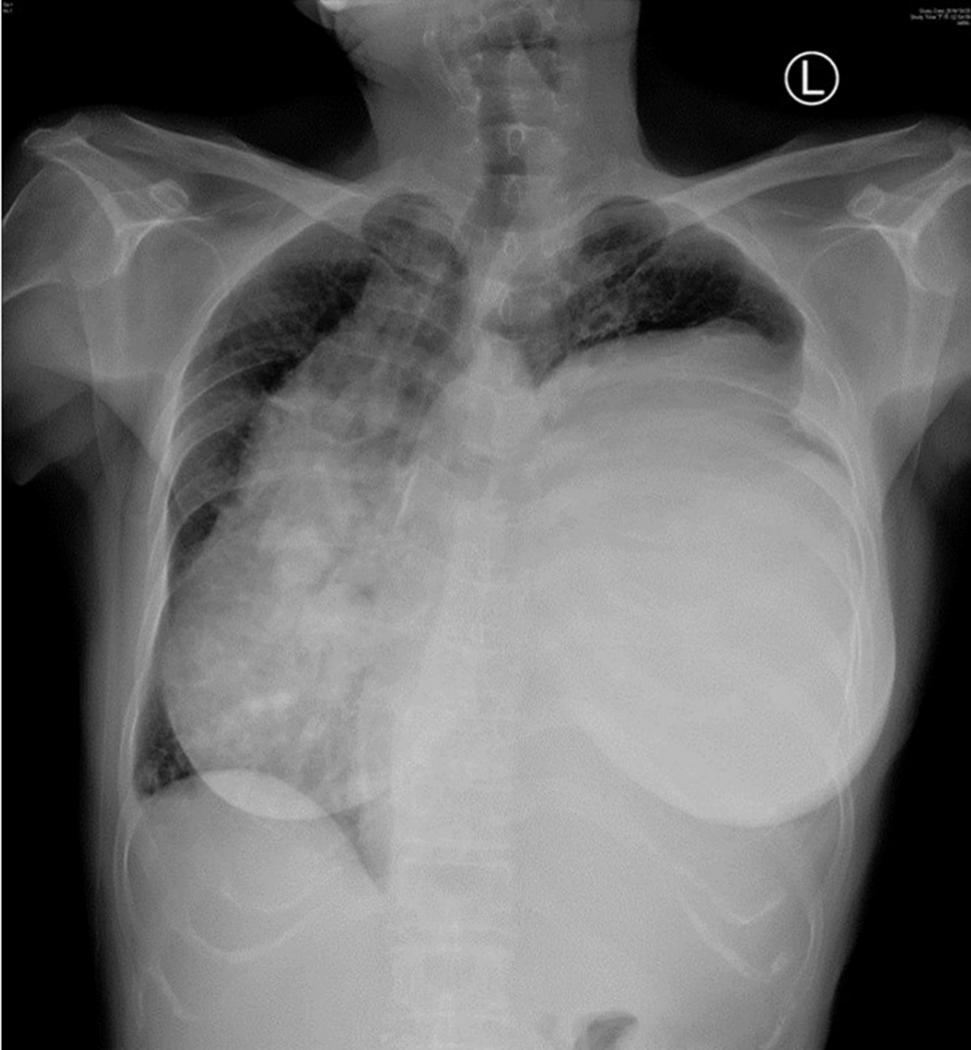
Plain radiograph of the chest
